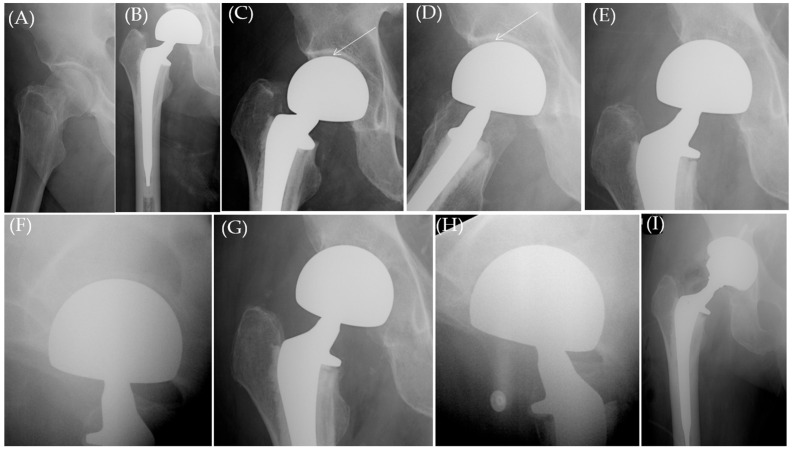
(A) Plain hip X-ray of a 76.5-year-old male (Case 10, Table 4) who sustained a right femoral neck fracture. (B–D) A 2-week postoperative plain hip radiograph showing bipolar HA with preserved joint space (white arrow), and (E,F) 11-week and (G,H) 22-week postoperative X-rays showing progressive loss of the joint space and advancement of the femoral head inside the acetabulum in the lateral view in comparison to the 2-week postoperative lateral radiograph (D). (I) Total hip arthroplasty performed 6 weeks later (28 weeks after the index HA surgery for debilitating groin pain).
